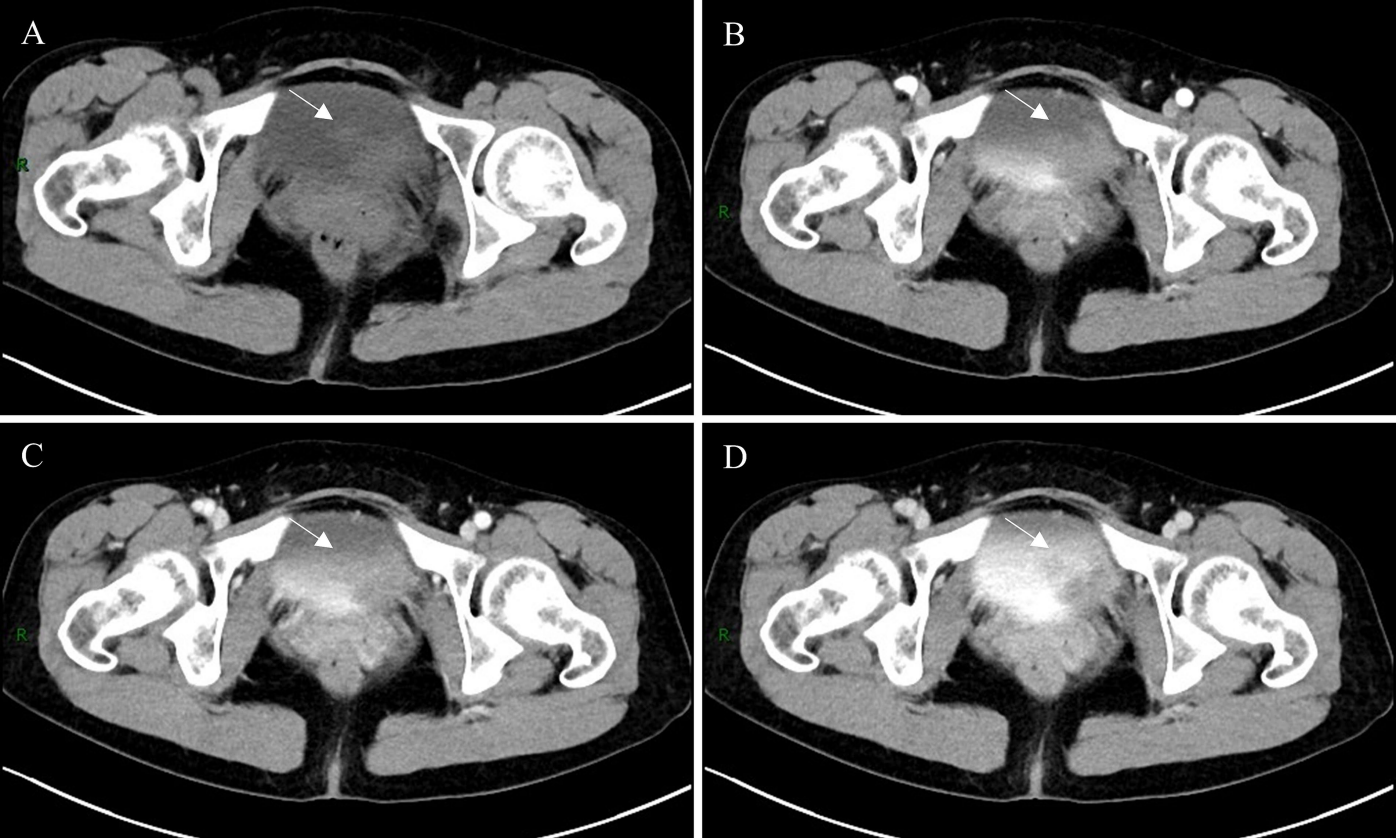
Non-contrast abdominal CT scan demonstrated an irregular soft tissue density lesion along the left lateral wall of the urinary bladder (A, arrow). The mass exhibited contrast enhancement during both arterial and venous phases (B,C, arrow), with subsequent excretory phase imaging revealing a focal filling defect in the corresponding region of the left lateral bladder wall (D, arrow).
